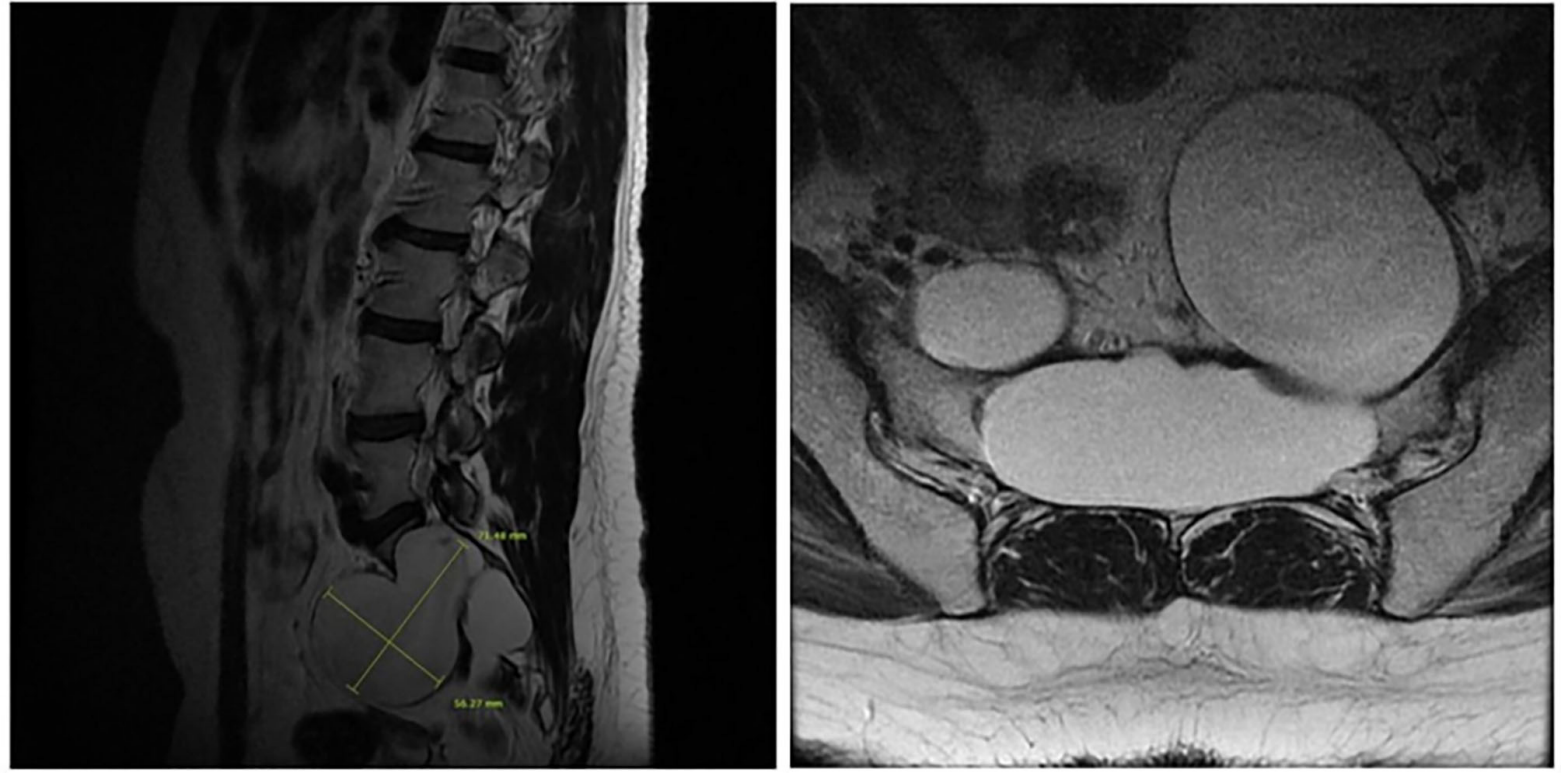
A sagittal T2-weighted image shows a 7.1 × 5.6 cm cyst that originated from the perineurium in the sacrum with extension to the pelvic cavity with bony erosion (left) and the sacrum on a transverse T2-weighted image (right) obtained 10 months after sclerotherapy.
